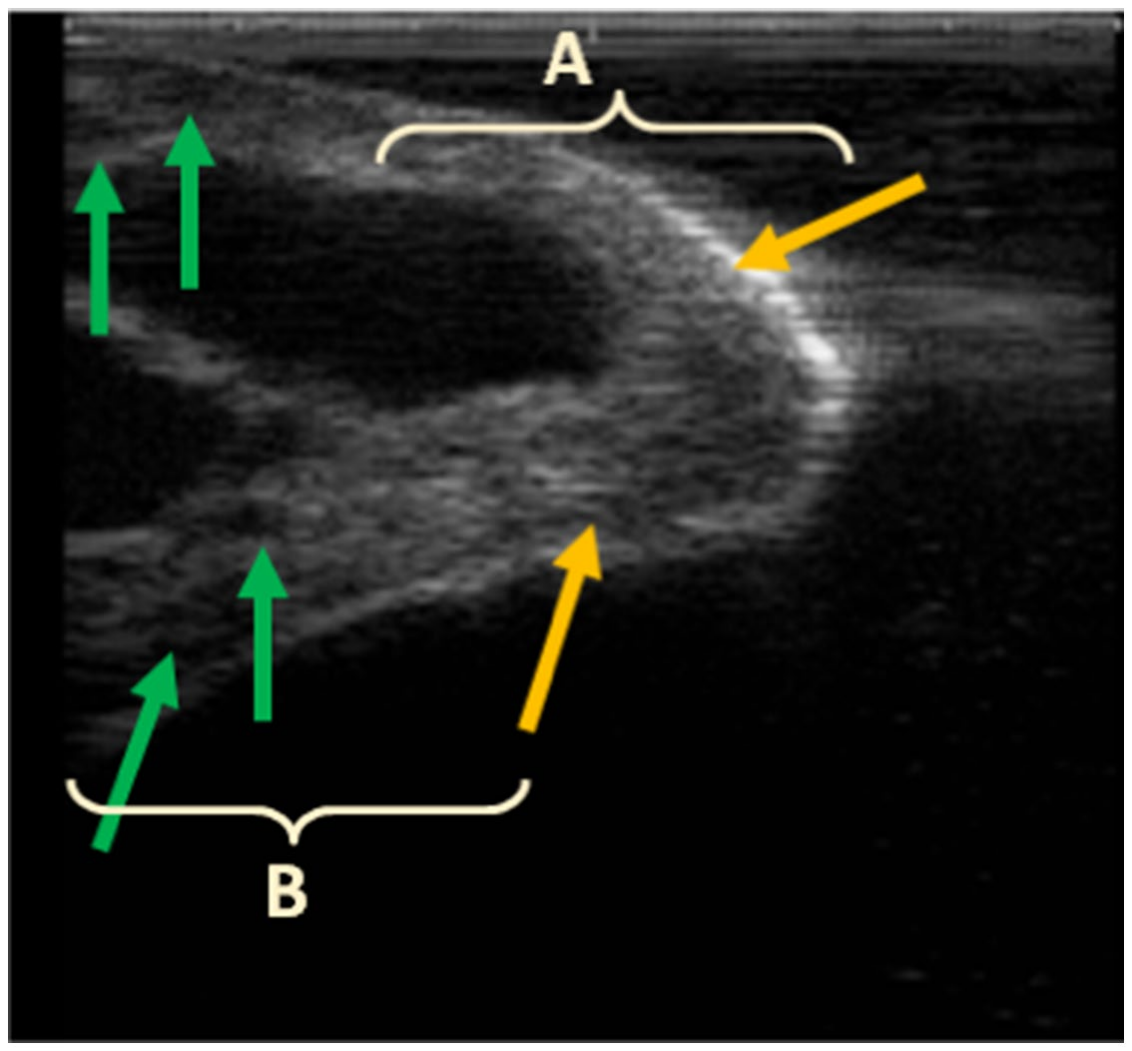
Post-milking ultrasound of the teat apex (A) and barrel (B); hyperechoic area at the tip of the teat (yellow arrows) and fluid accumulation in the teat barrel, seen as small hypoechoic areas in the teat wall (green arrows).
